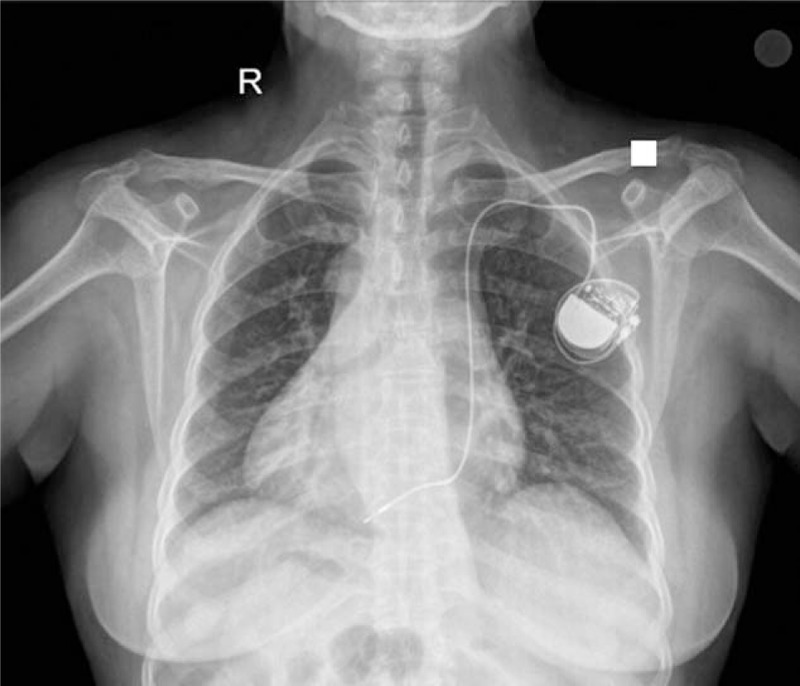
Chest X-ray demonstrating the correct placement of the VVI pacemaker.
